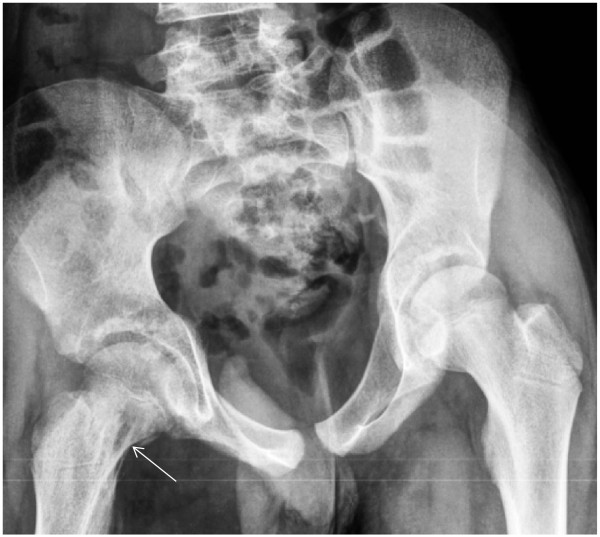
Radiograph of the pelvis showing an osteolytic lesion in the meta-diaphyseal region of the right femur with cortical discontinuity and increased volume and soft tissue density in the intra-articular projection (arrow), which is consistent with the existence of an effusion.
